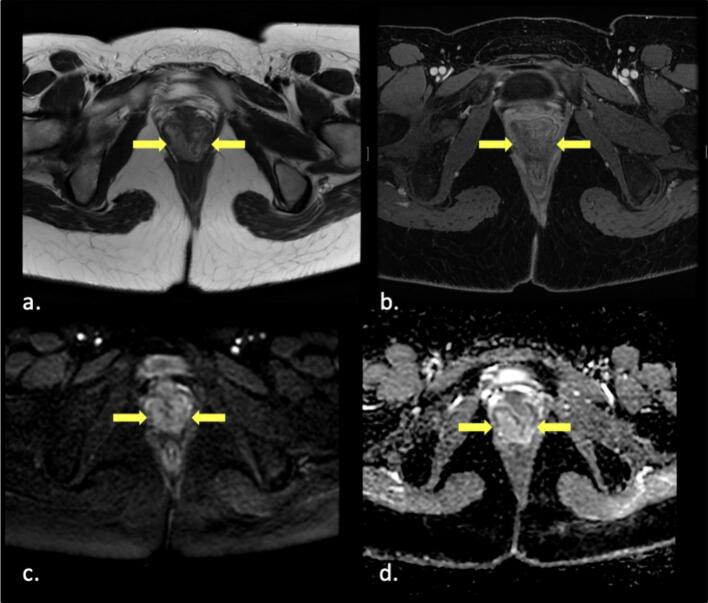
(a.) Axial T2WI at the level of distal vagina showing laminated appearance of posterior vaginal mass displacing the collapsed vaginal lumen anteriorly. (b.) corresponding T1WI post IV contrast showing minimal enhancement. (c and d) corresponding DWI showing no diffusion restriction.
